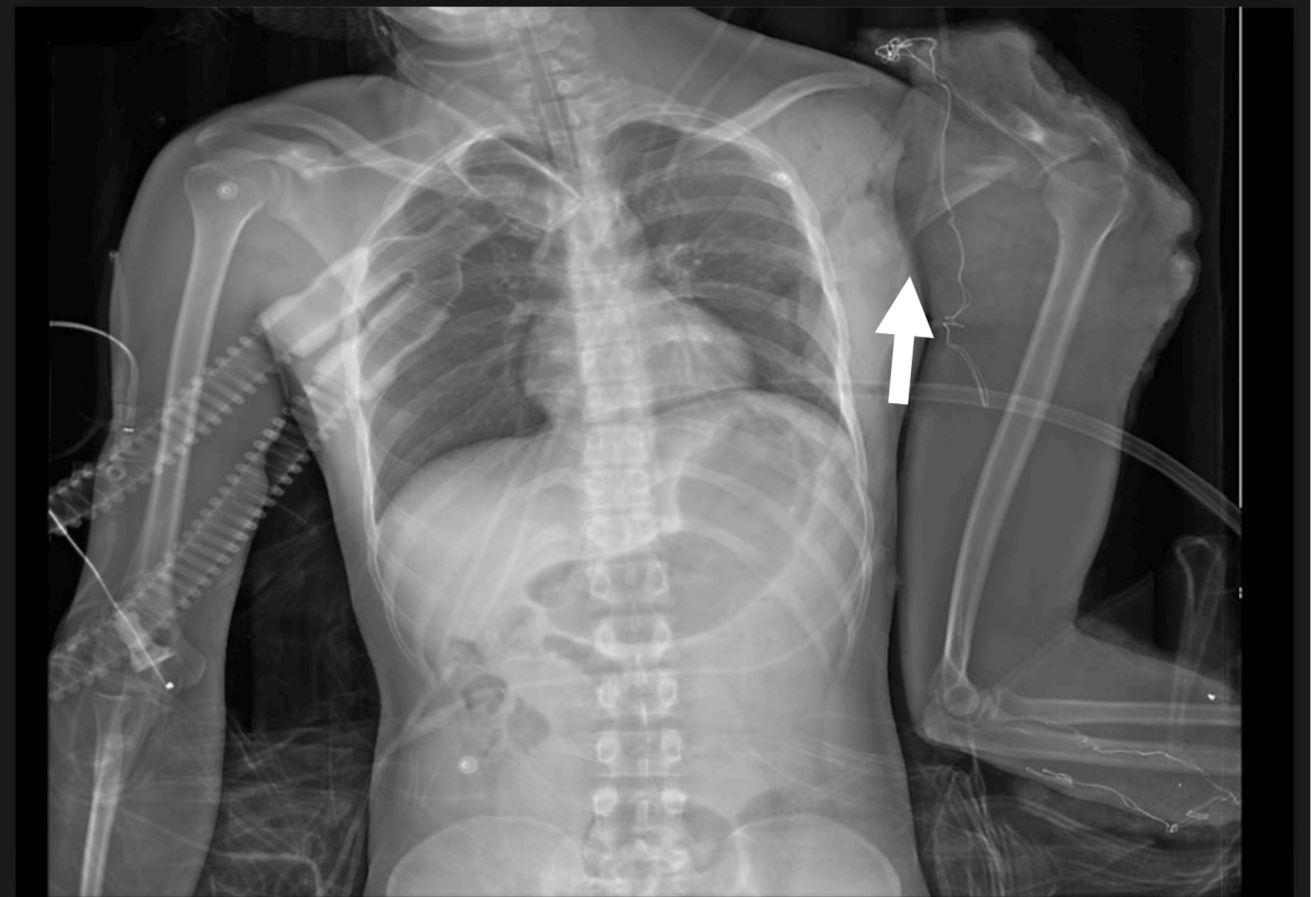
Radiograph (Lodox) on admission showing lateral displacement of the left scapula from the thoracic wall (arrow), consistent with open STD. STD: Scapulothoracic dissociation
